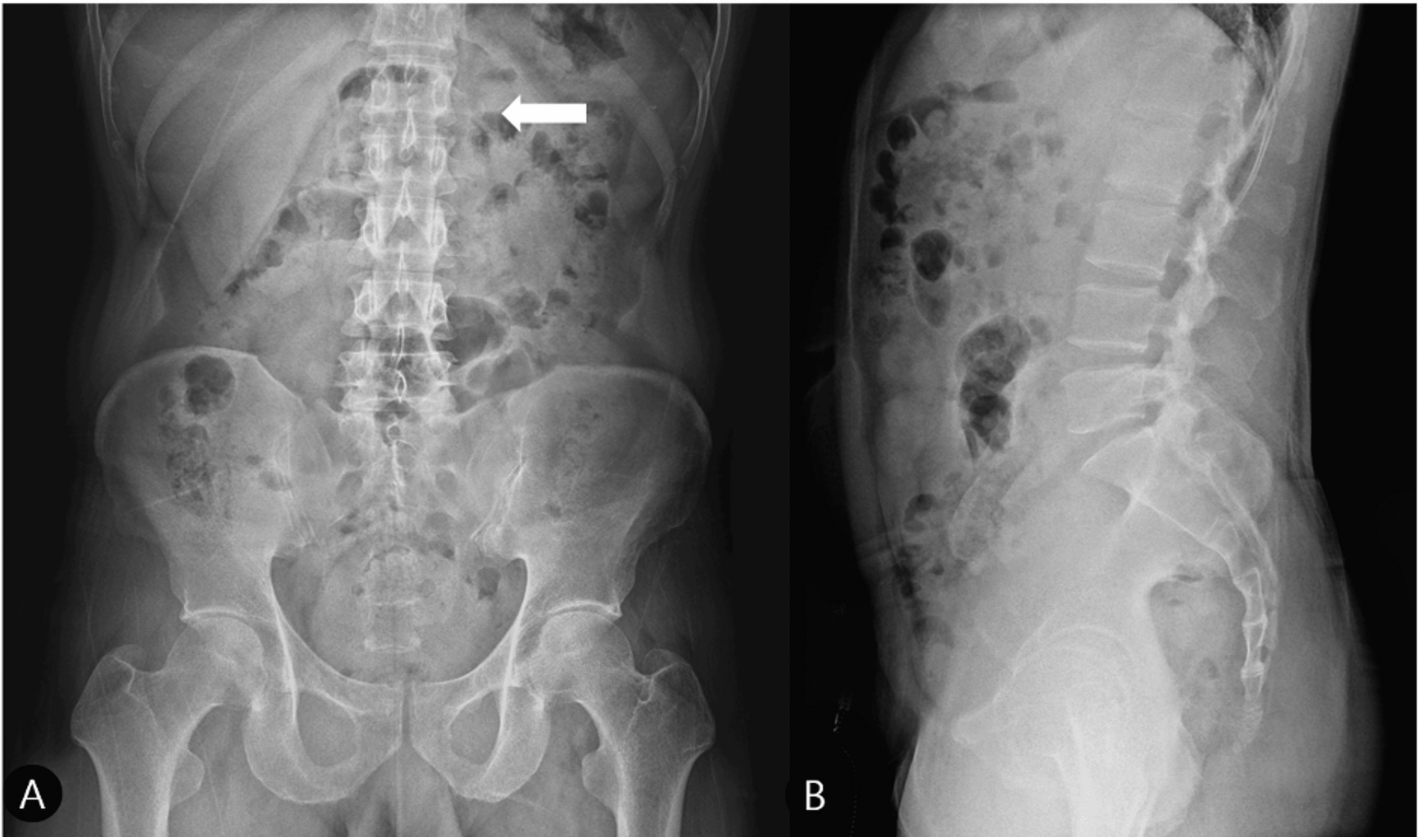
One-month follow-up lumbar X-ray One-month follow-up lumbar X-ray (AP view [A], lateral view [B]). Bridging callus and improved alignment at the left L2 transverse process are observed (solid arrow). Notable improvement in spinous process alignment is evident compared to baseline.
